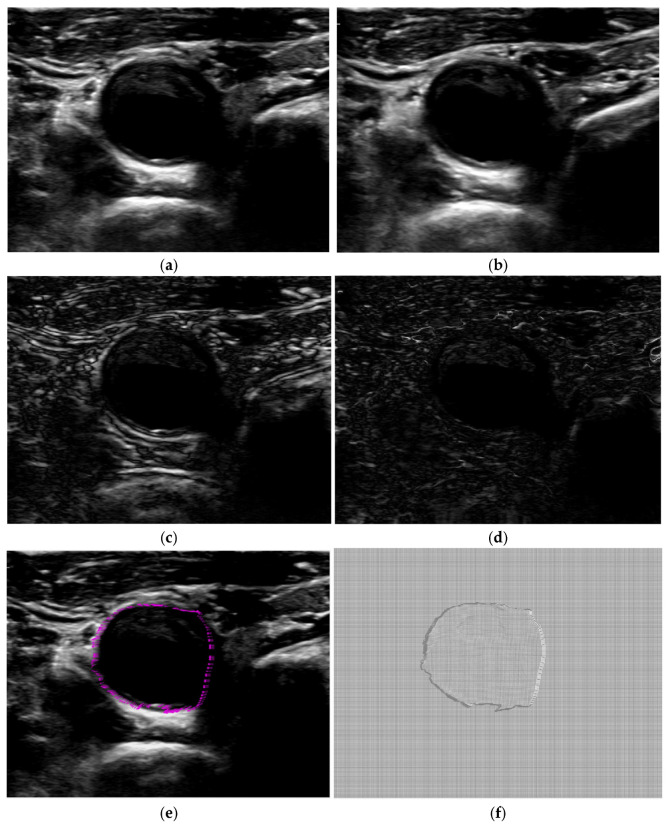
In vivo carotid artery cross-section ultrasound images: (a) the source image, (b) the target image, (c) the difference image between the source and target image, (d) the results obtained by the proposed algorithm, (e) the displacement vector from the boundary in a (red arrows), and (f) the deformation field.
